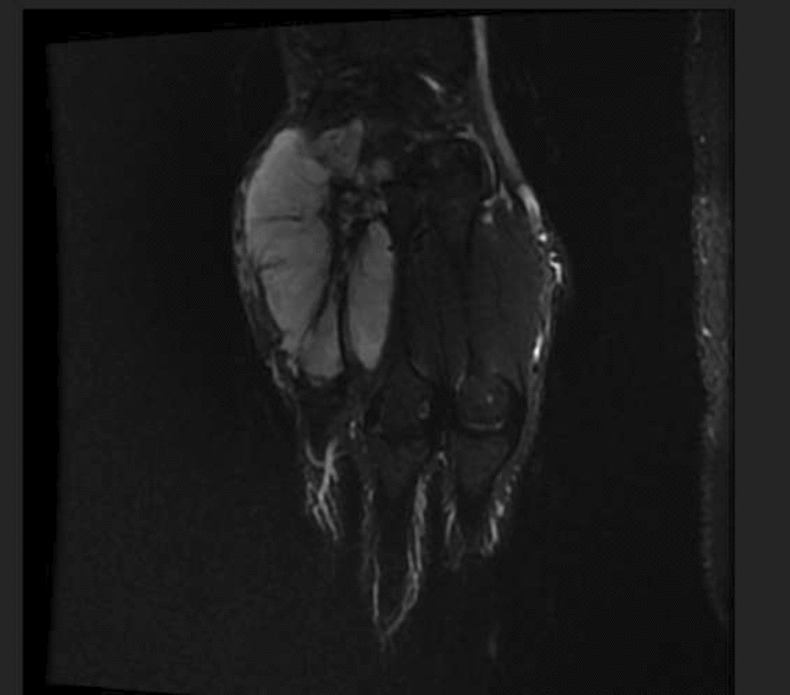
Magnetic resonance image of the right hand showing a heterogeneous mass-like t2 signal intensity lesion encasing the second metacarpal with pressure effect over flexor and extensor tendons
